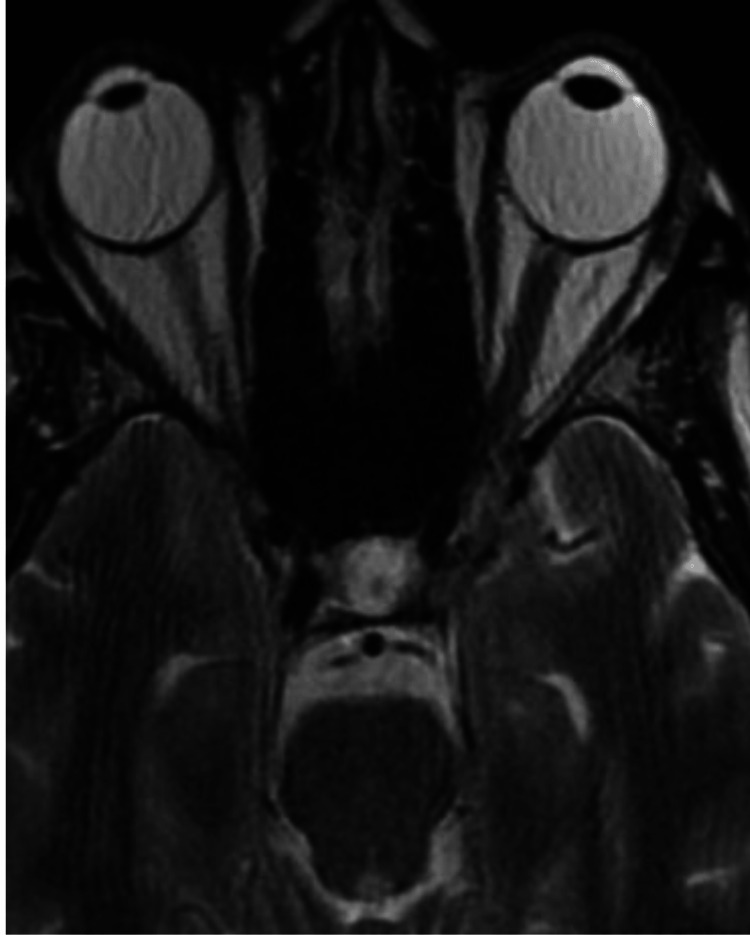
MRI of the brain (with contrast) MRI orbits were unremarkable for flattening of the posterior globe, optic nerve atrophy, or demyelination.
